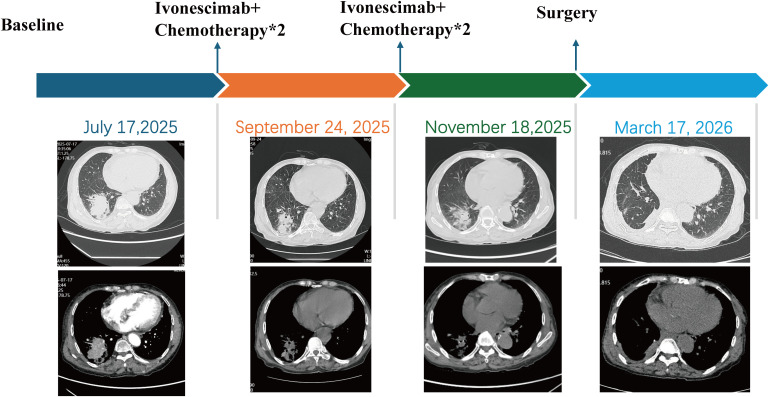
Timeline of treatment course and radiological evaluations from July 2025 to March 2026. CT, computed tomography.
